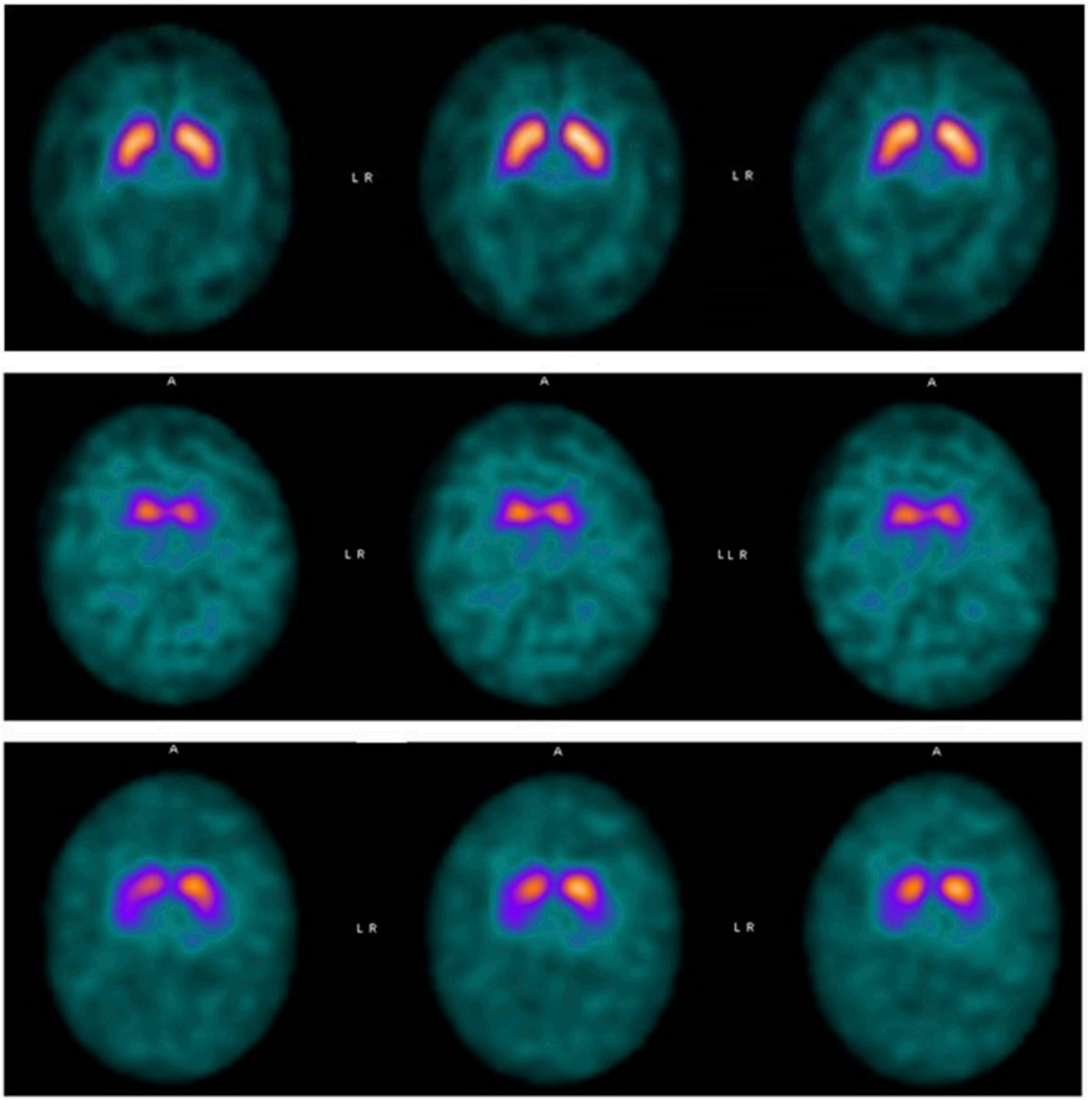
Top panel: DaTSCAN SPECT normal. Middle panel: Patient 1: ligand binding in both putamina was almost absent. The uptake was also markedly and symmetrically reduced in the caudate nucleus bilaterally. Bottom panel: Patient 2: DaTSCAN SPECT showed considerably decreased ligand binding in both putamina. Initial uptake reduction was observed also in the right caudate.
